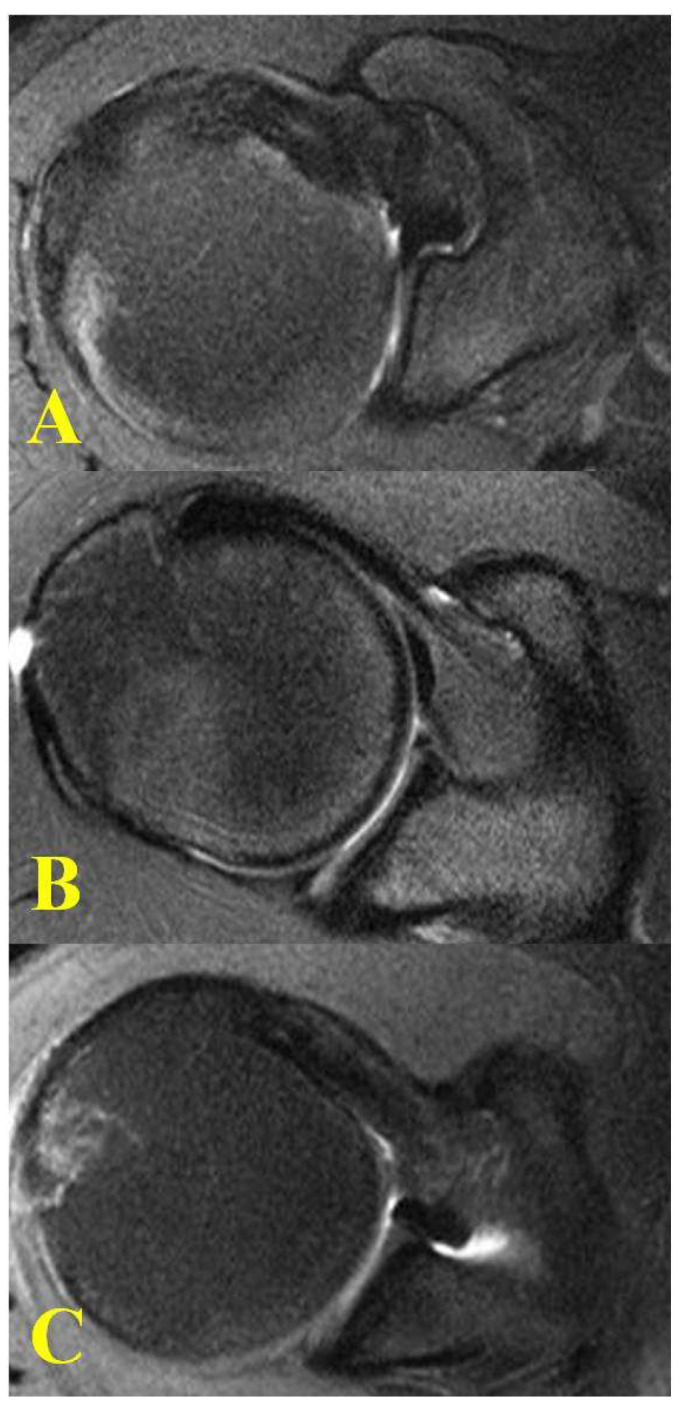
Coracoid Morphology on axial MRI of shoulder: (A) Curved; (B) Hook; (C) Flat.
